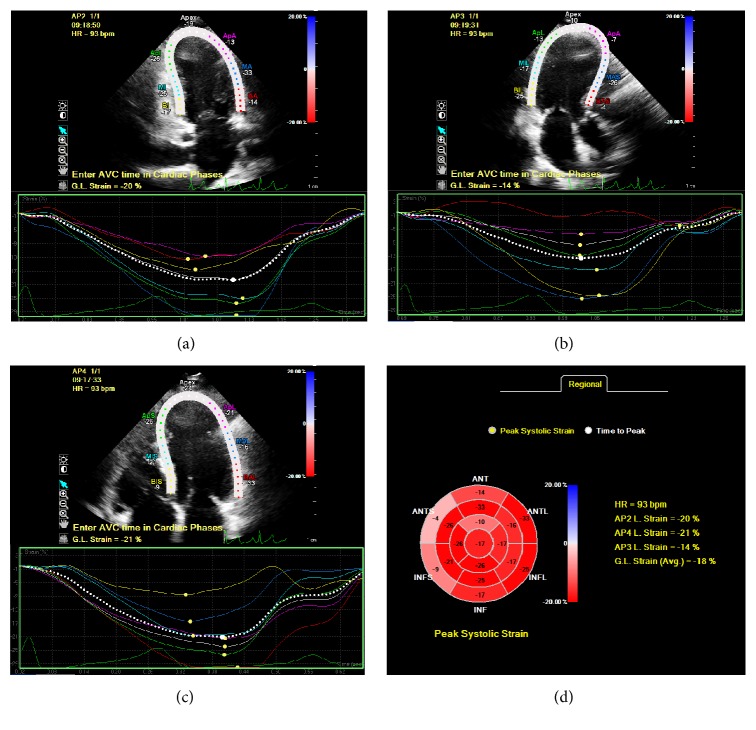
Peak systolic strain assessment using speckle tracking echocardiography. (a) Two-chamber left ventricular strain. (b) Three-chamber left ventricular strain assessment. (c) Four-chamber left ventricular strain. (d) Global and regional strain assessments generated from 2-chamber, 3-chamber, and 4-chamber views.
